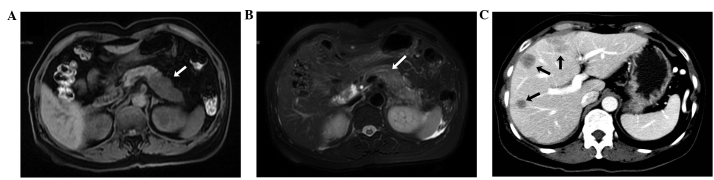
Magnetic resonance cholangiopancreatography imaging findings and diagnosis for (A) tumor in body and tail of pancreas and (B) interruption of the pancreatic duct, and (C) computed tomography imaging findings of the liver metastasis.
